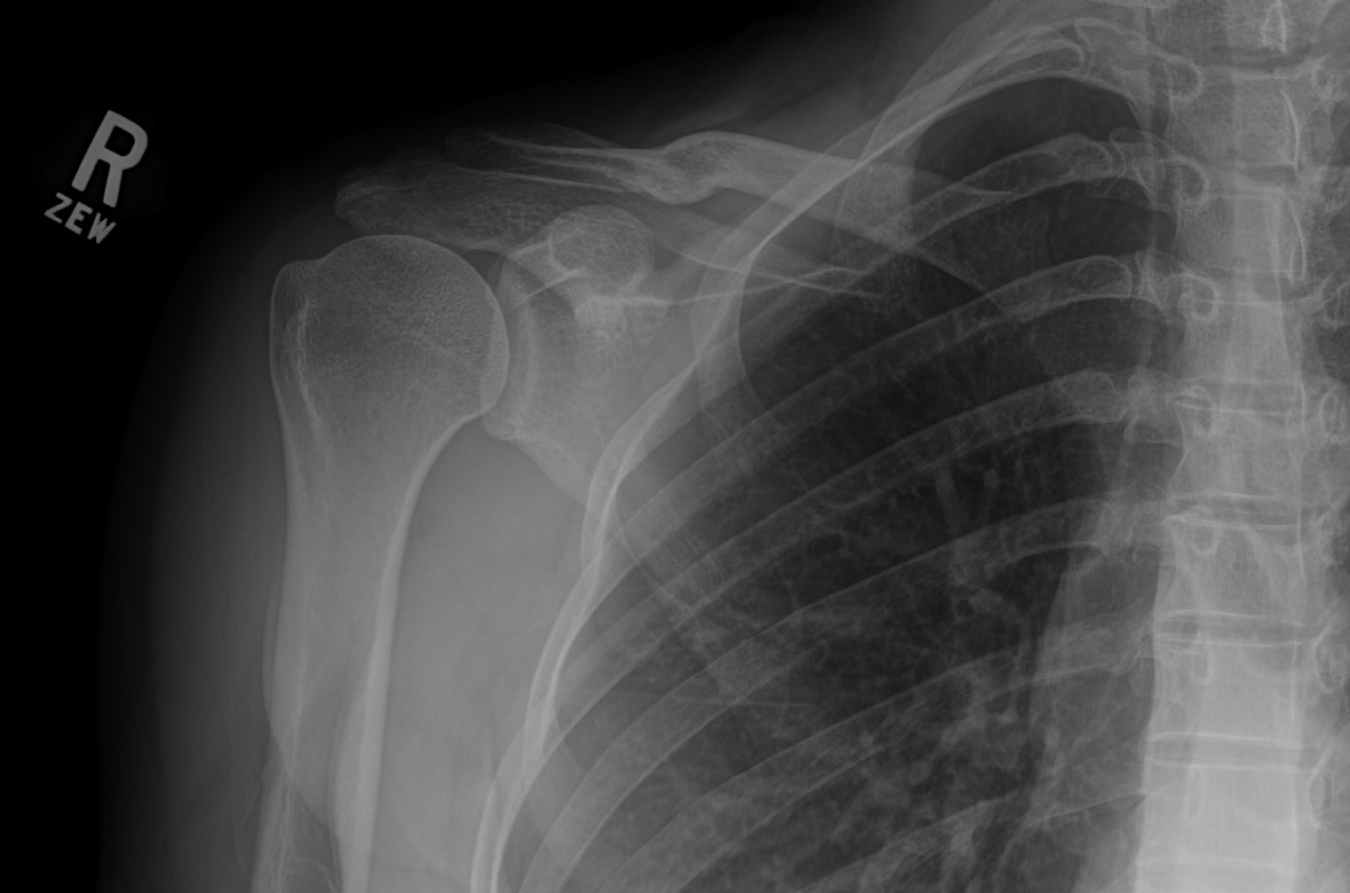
Preoperative axial radiograph of *Right* clavicle of patient at 26 years old.
